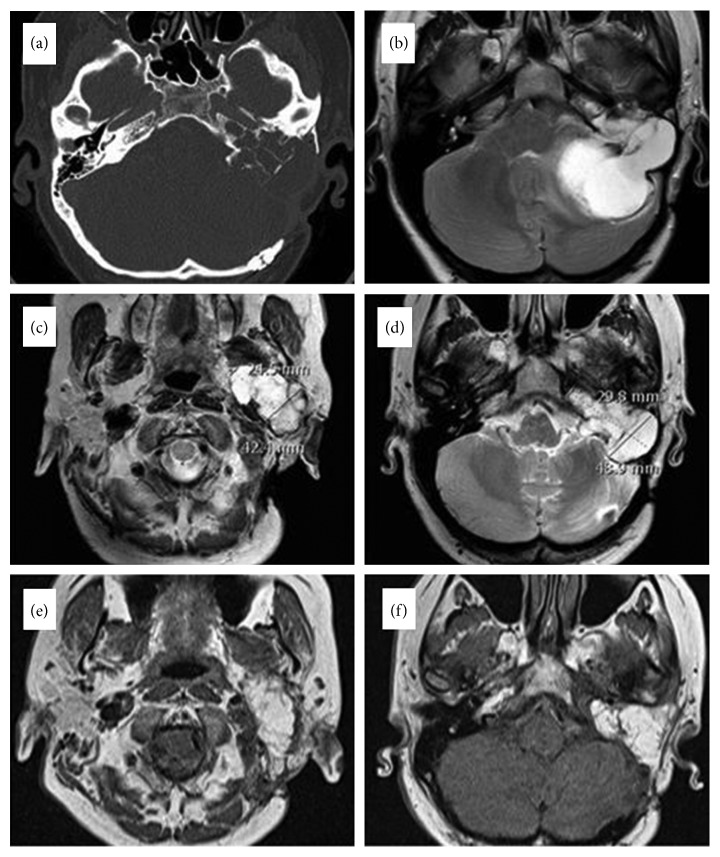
Pre- and postoperative CT and MRI scans of Case 2. (a) Preoperative CT scan showing calcifications within the tumour. (b) Preoperative MRI showing a large arachnoid cyst posteriorly to the tumour at the right cerebellopontine angle. Note remarkable compression of the cerebellum and brainstem caused by the cyst. (c, d) Preoperative MRI scans showing tumour masses in the right parapharyngeal space and at the projection of the temporal bone, respectively. (e, f) Postoperative MRI scans showing fat tissue at the right parapharyngeal space and at the projection of the temporal bone, respectively.
